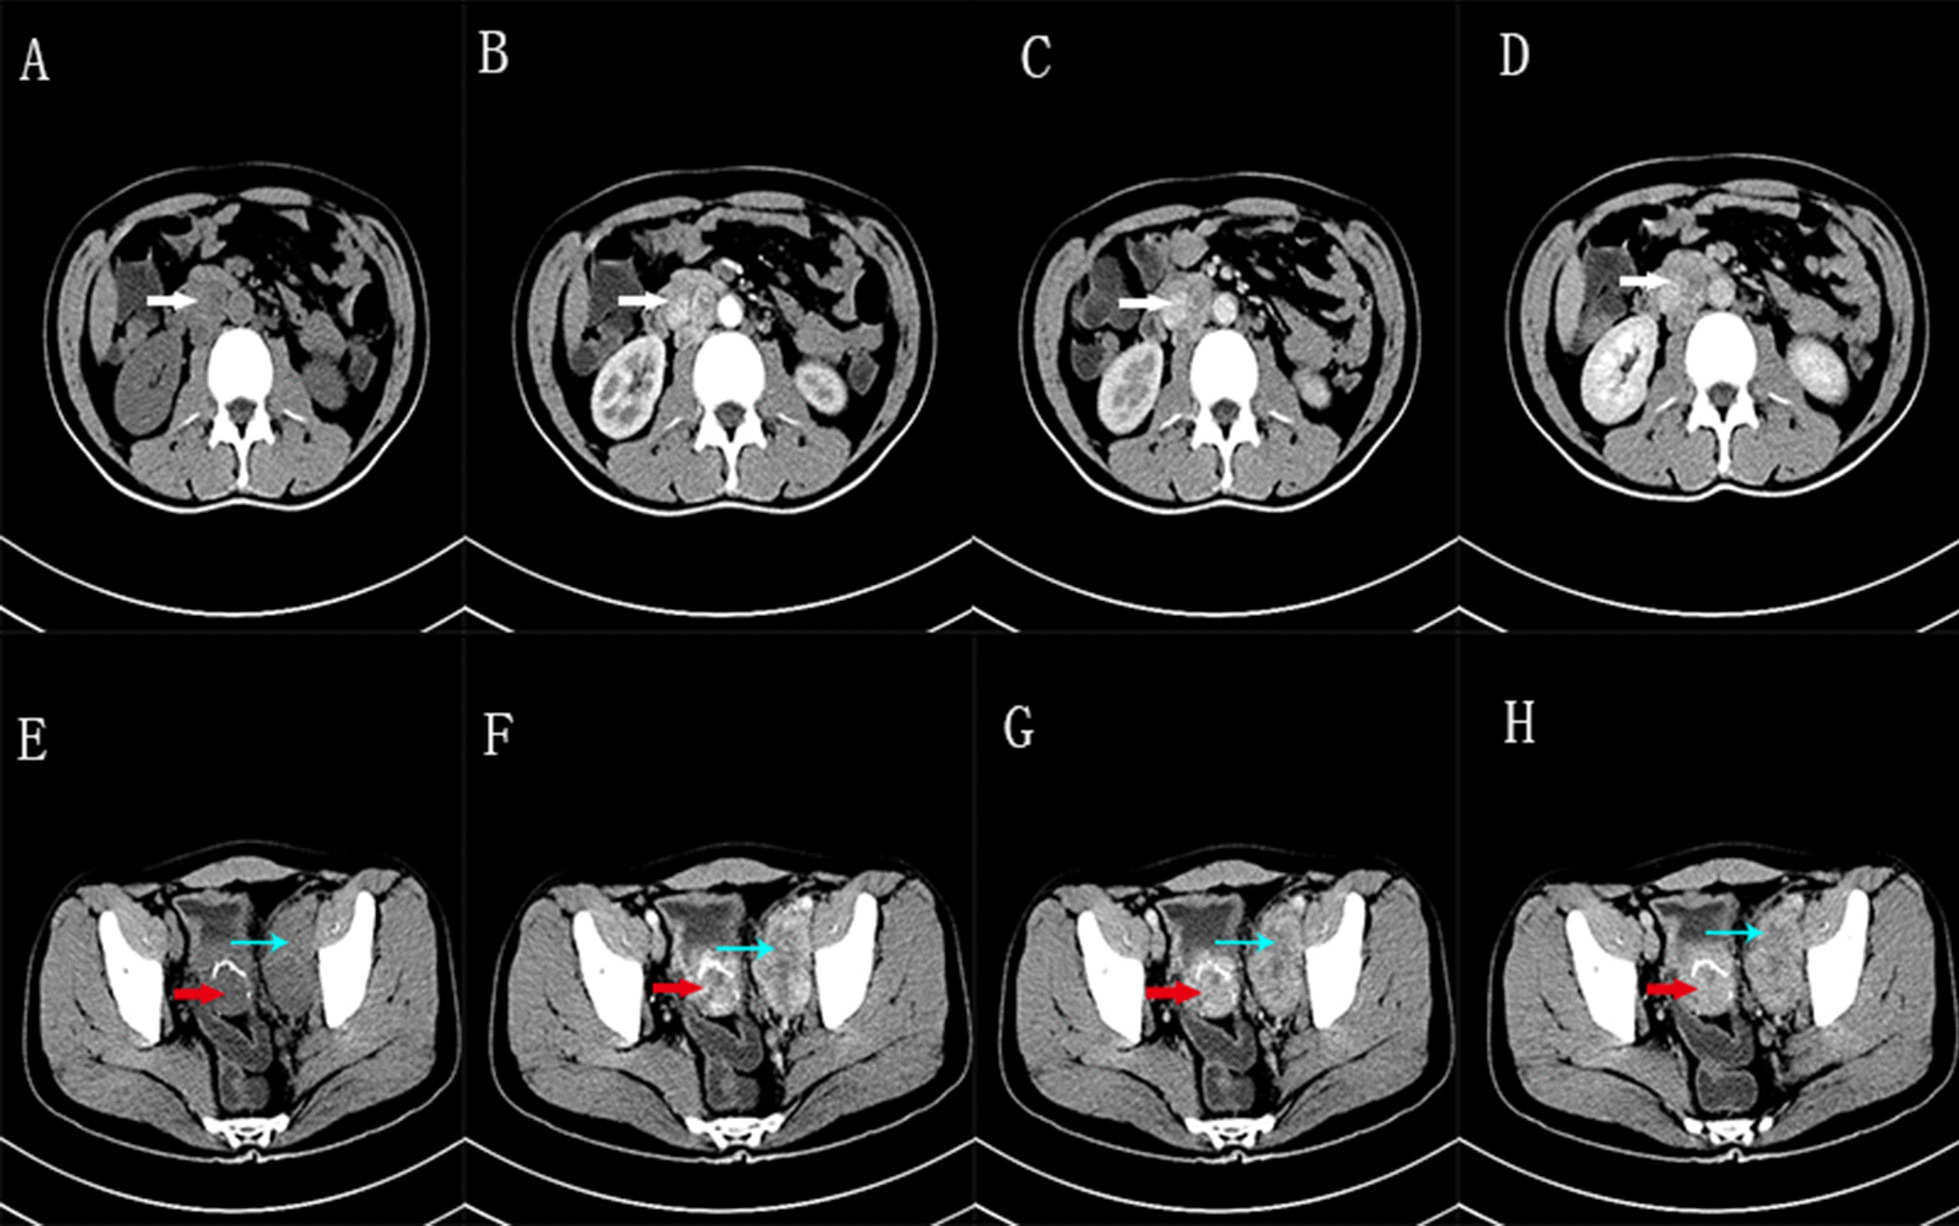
Upper, lower abdomen, pelvic cavity enhanced CT. A and E Plain scan; B and F arterial phase; C and G venous phase; D and H delayed phase. Blue and white arrows indicate enlarged lymph nodes. Red arrows indicate the mass shadow of the posterior wall of the bladder
